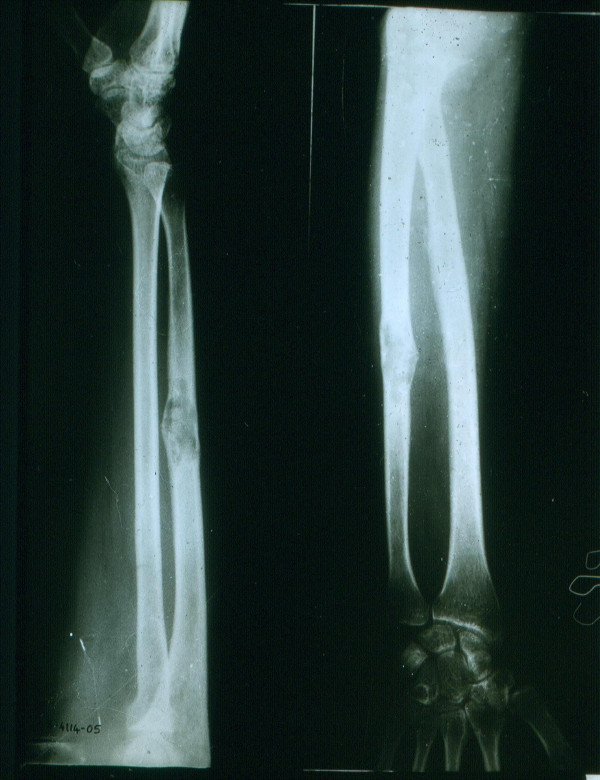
X-ray of postero-anterior and lateral view of right forearm showing an expansile lytic destructive lesion involving mid one-third of diaphysis of ulna, with ill-defined zone of transition.
